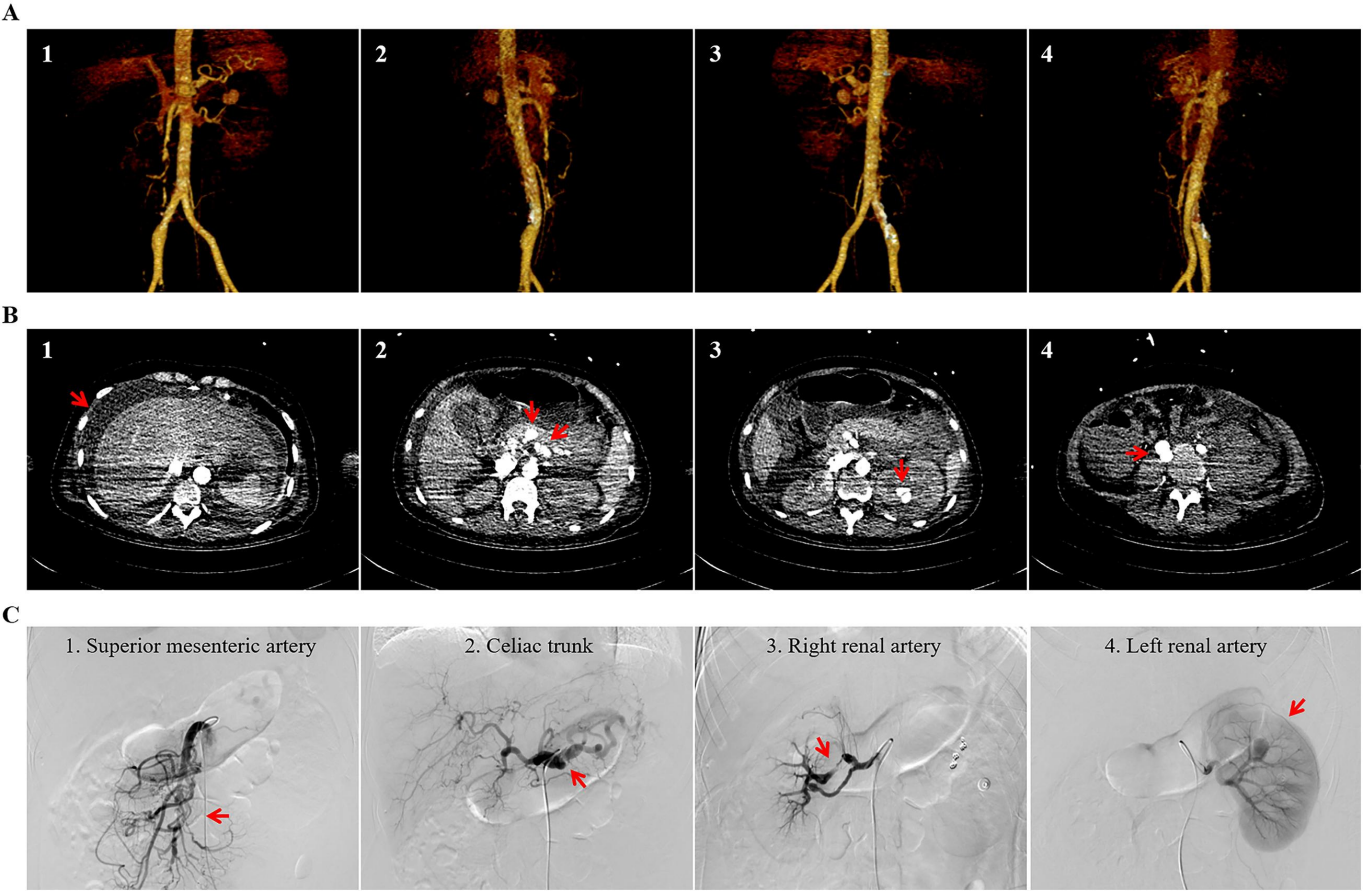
Fibromuscular dysplasia led to renal artery rupture with the involvement of the celiac trunk, splenic, bilateral renal, and superior mesenteric arteries. A CT angiography showed a left renal intraparenchymal hematoma, a common celiac artery aneurysm, and true or false aneurysms alternating with stenosis or occlusion and arterial tortuosity in the celiac trunk, splenic, bilateral renal, and superior mesenteric arteries. The three-dimensional CT angiographic images are in the anterior (A1), right (A2), posterior (A3), and left (A4) views. The cross-sectional CT images indicate perihepatic effusion (B1), aneurysm and stenosis in the celiac trunk (B2), renal aneurysm rupture (B3), and aneurysm in the right common iliac artery (B4). Angiographic images of the visceral branch arteries were obtained. The typical “string-of-beads” sign was consistent with fibromuscular dysplasia of the superior mesenteric artery (C1). Angiography shows aneurysms alternating with stenoses in the celiac trunk (C2) and right renal artery (C3). Left renal aneurysm rupture was proved by invasive angiography (C4) (indicated by arrows).
